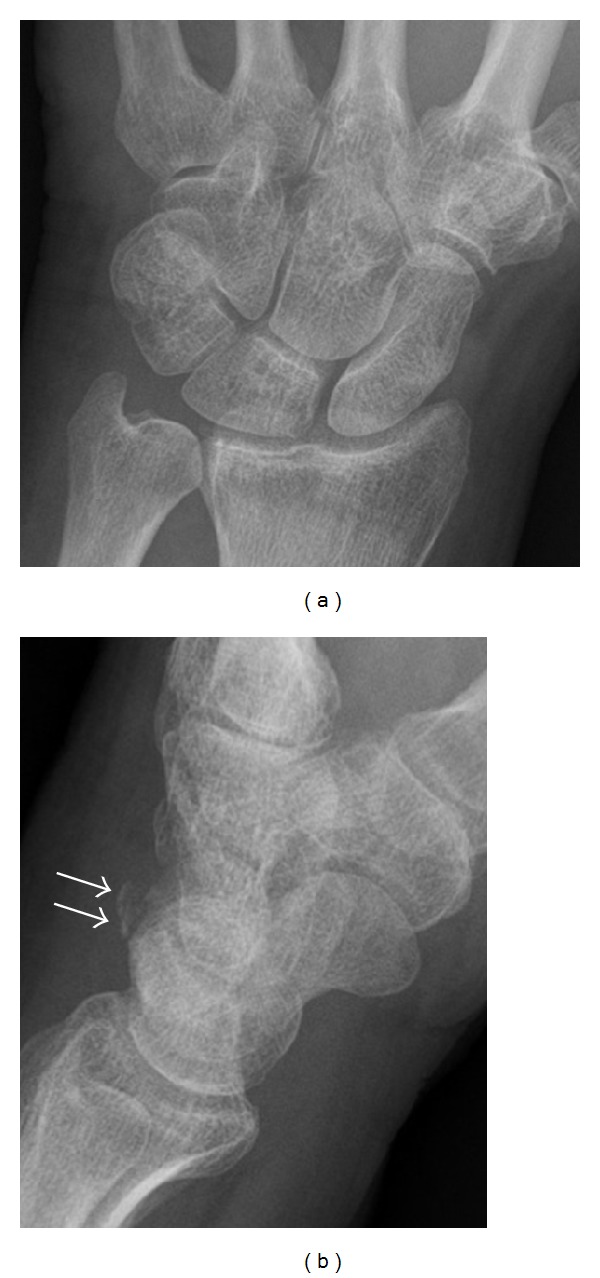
Dorsal triquetral fracture of the left wrist in a 30-year-old man after a trauma. Anteroposterior radiograph shows a normal appearance. (b) Lateral radiograph of the same wrist demonstrates a chip fracture off the dorsal aspect of the triquetrum (arrow).
